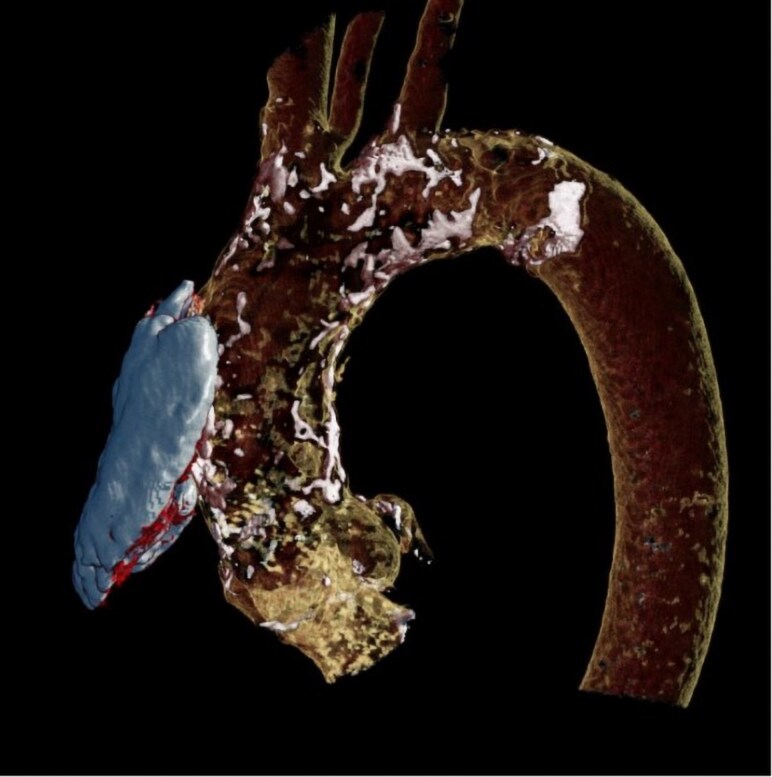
A 66-year-old female with a history of mantle radiotherapy for Hodgkin’s lymphoma underwent computed tomography angiography for planning a transcatheter aortic valve implantation as a result of radiation induced aortic valve stenosis. The imaging reveals heavily calcified anterior mediastinal lymph nodes (highlighted in blue) and diffuse calcification (highlighted in white) of the ascending aorta and aortic arch, indicative of radiation-induced aortitis.
